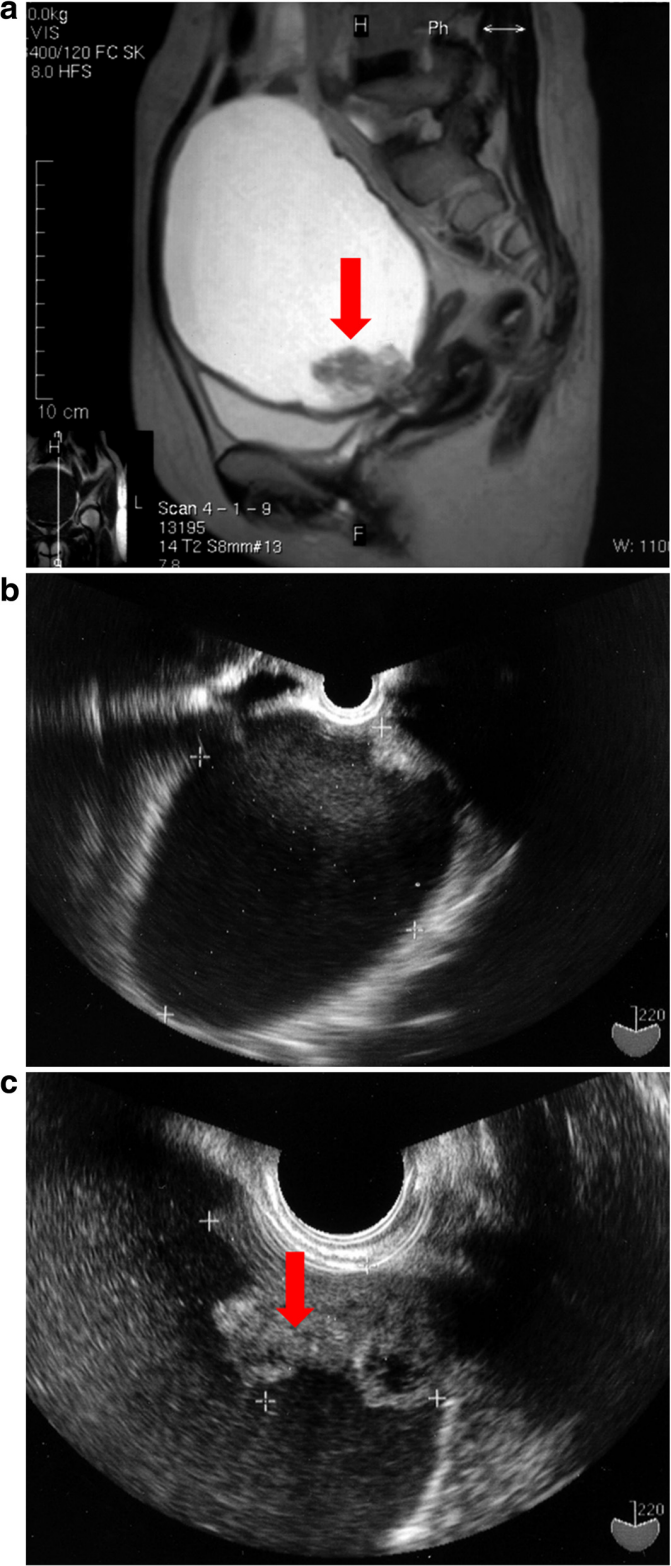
a Sagittal section of T2 MRI revealed a unilocular cystic tumor measuring 14 cm in diameter. There is a papillary nodule (red allow) in the tumor wall. b Transvaginal ultrasound revealed a unilocular cystic tumor measuring 14 cm in diameter. c Transvaginal ultrasound revealed a papillary nodule measuring 4 cm in the tumor wall
